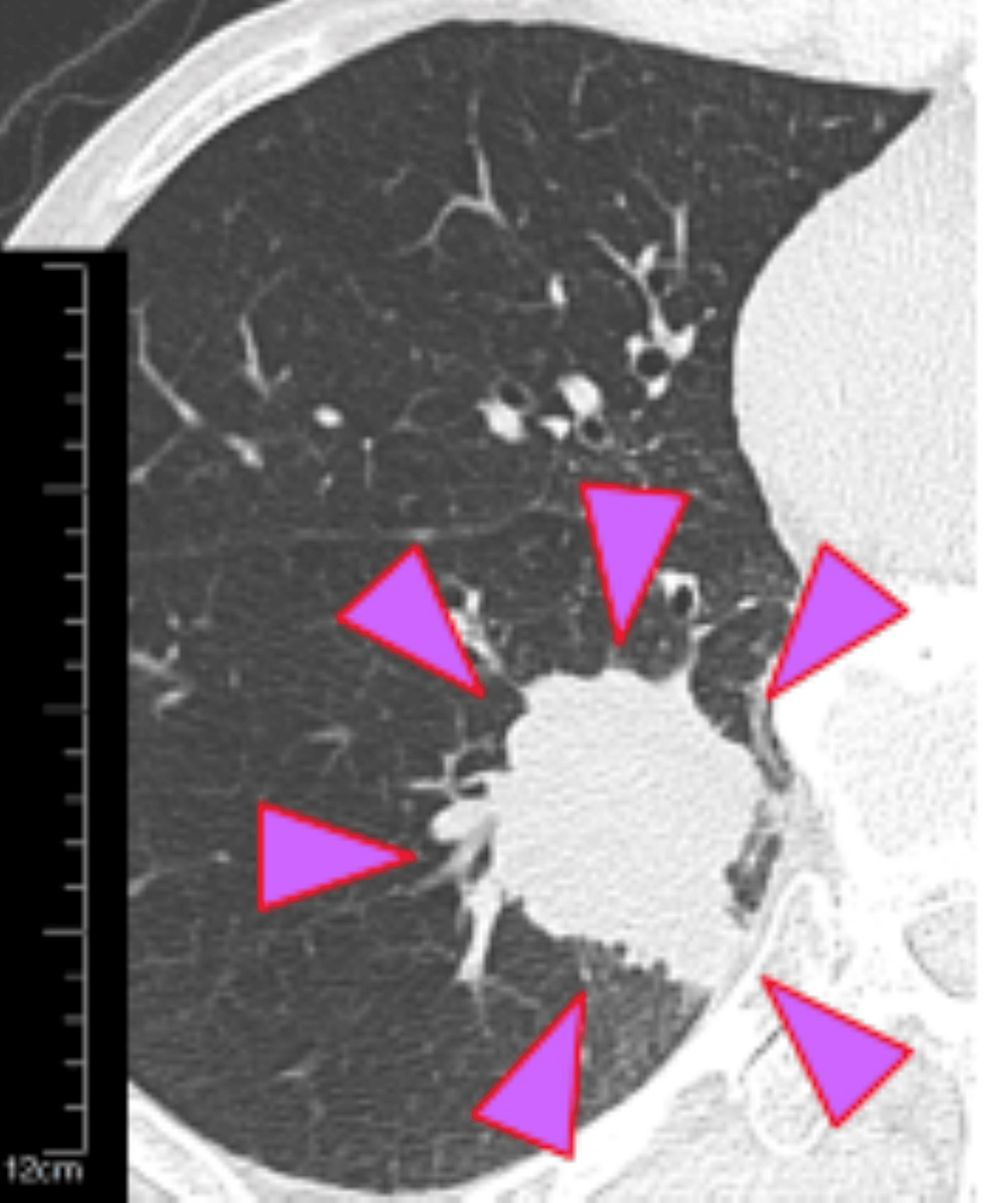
Chest CT of the patient showing a right lower lobe lung mass A tumor with a total lesion diameter of 3.8 cm and a solid component diameter of 3.8 cm was found in S9-10 of the right lower lobe CT: computed tomography
